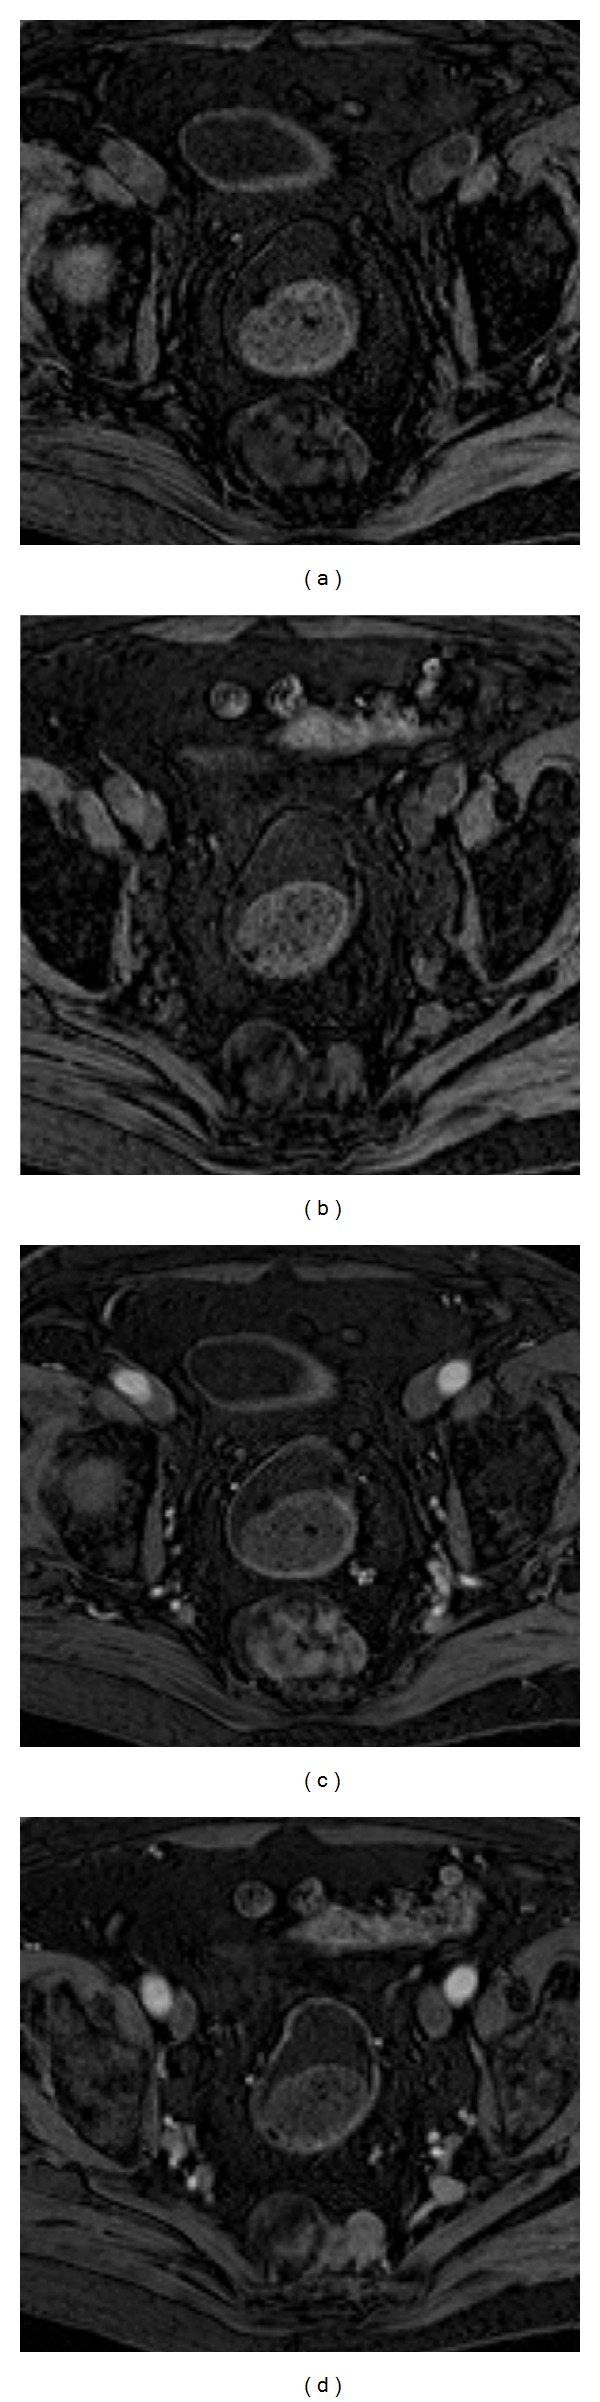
84-year-old male with presacral myelolipoma. Postcontrast T1-weighted fat-suppressed axial images at a higher (a) and lower (b) level of the mass demonstrated avid gadolinium uptake of the solid hematopoietic components of the lesion.
